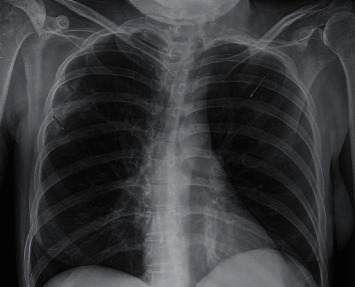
Chest radiograph demonstrating erosive changes of the fifth and sixth ribs.
